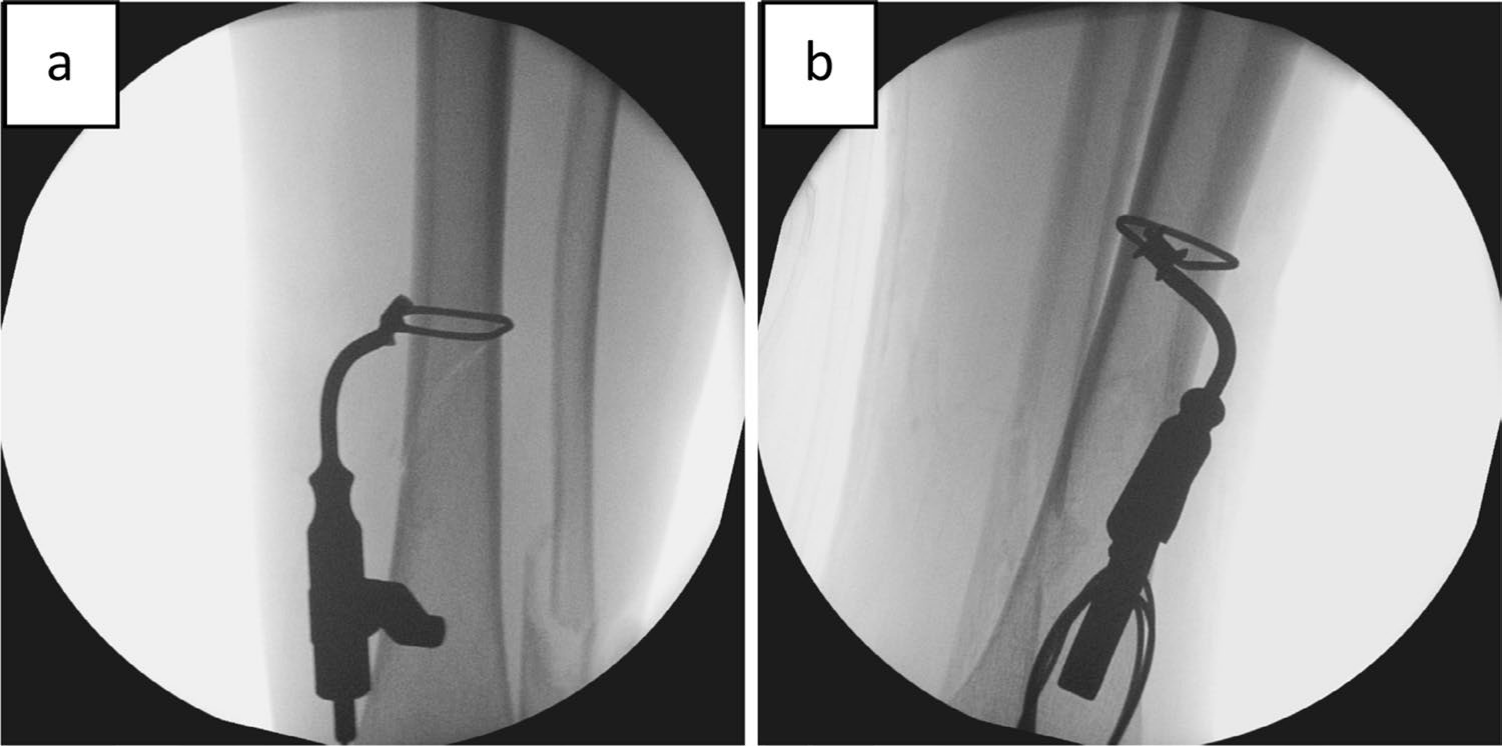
After tightening of the cerclage, the tibial fracture is reduced anatomically (X-ray in ap a and lateral b view)
